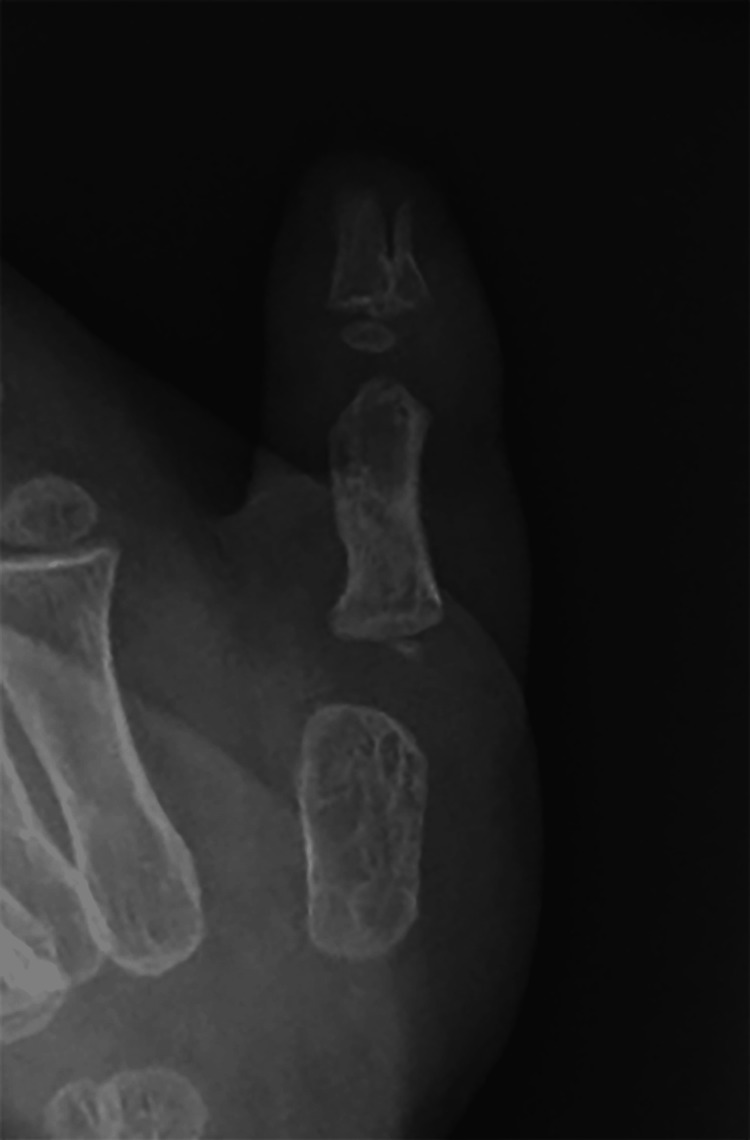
Radiographic image obtained 12 months after surgery
